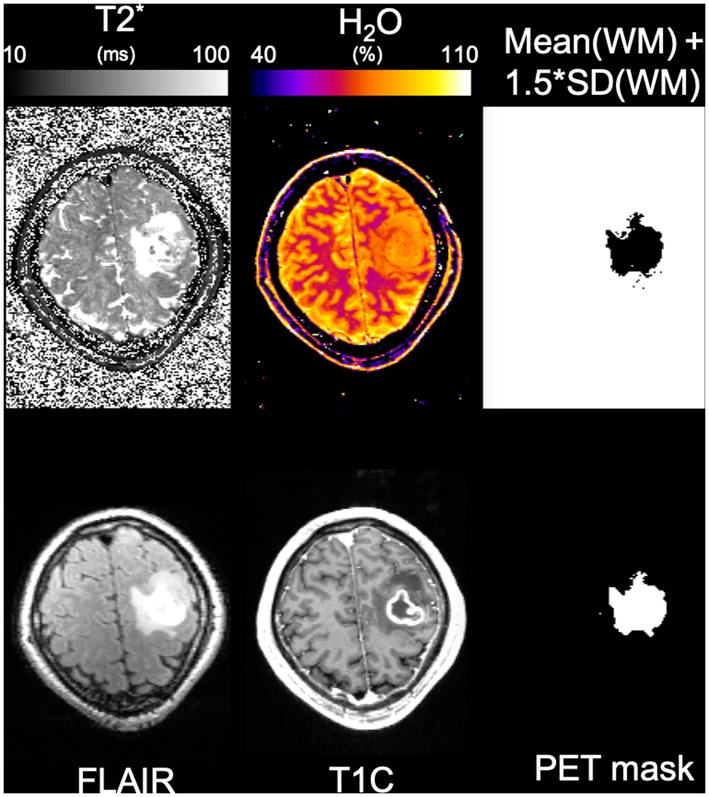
Results from the tumor patient. The same representative slice from the lesioned region is shown in each panel. The panels shown are, from left to right, top to bottom, T_2_*, water content, binary map obtained from the mean water content in WM plus standard deviation in WM, FLAIR, MP-RAGE post-contrast, and active brain mask obtained from PET.
